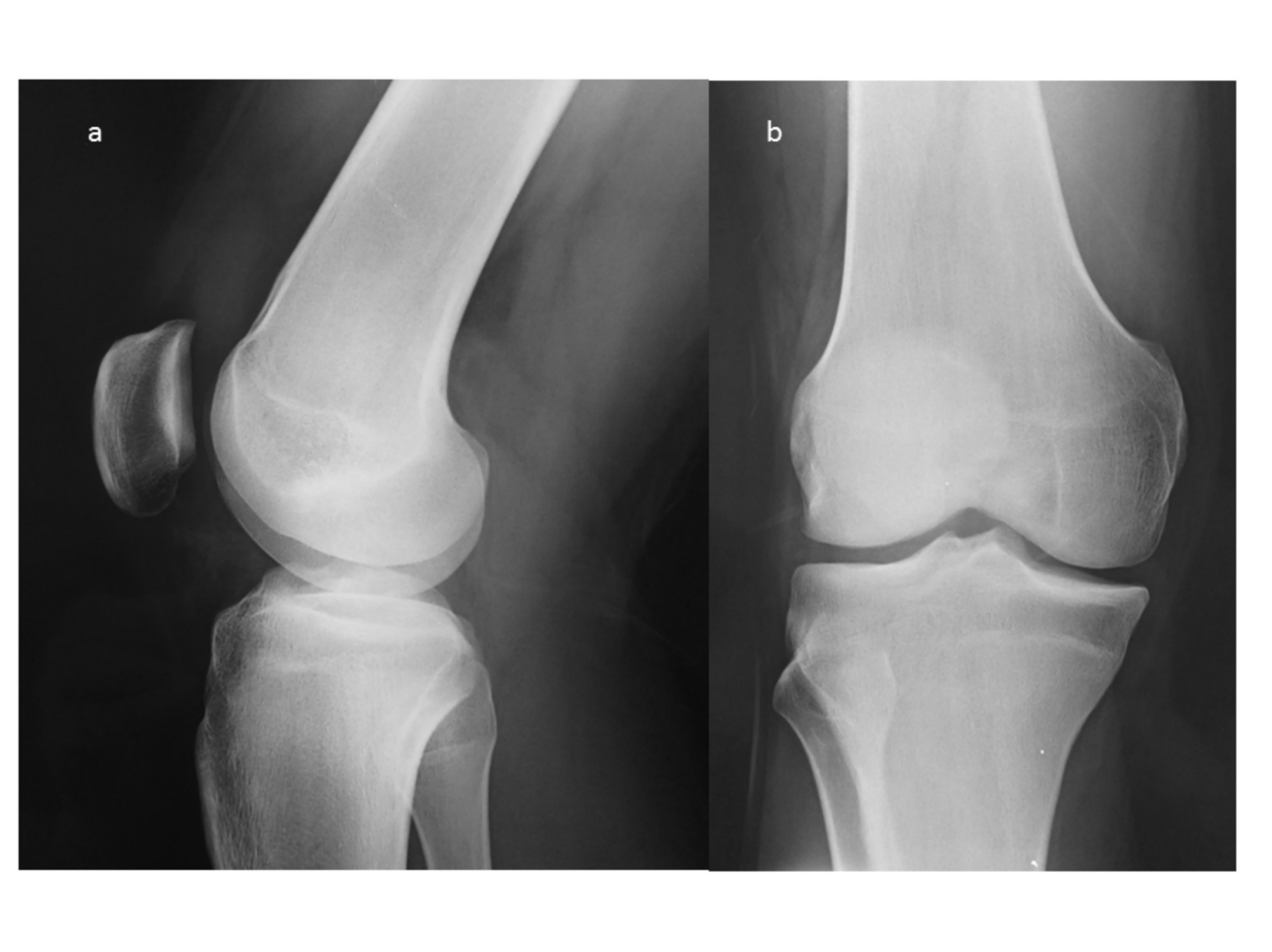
Knee x-ray of the patient a. Sagittal view, b. Coronal view
